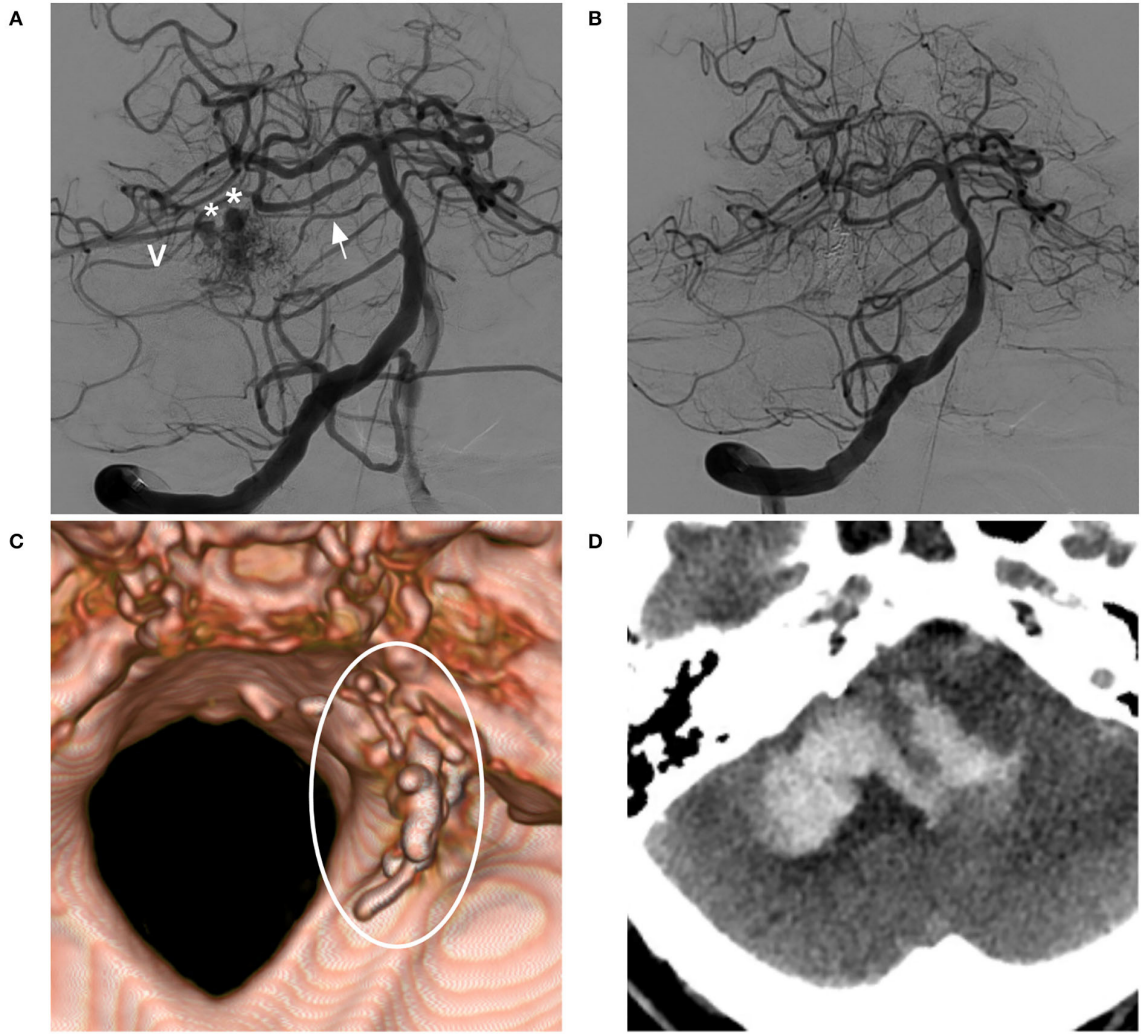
BAVM with post-operative venous rupture (case 13). (A) Angiogram of the VA showing the BAVM supplied by the SCA and pontine branch (arrow), with two venous aneurysms (asterisks) on the draining vein (V). (B) Post-operative angiogram showing complete embolization of the BAVM and not showing the draining vein. (C) Post-operative CT reconstruction showing Onyx casting (circle). (D) Post-operative CT showing venous hemorrhage away from the nidus. BAVM, brain arteriovenous malformation; CT, computed tomography; VA, vertebral artery.
